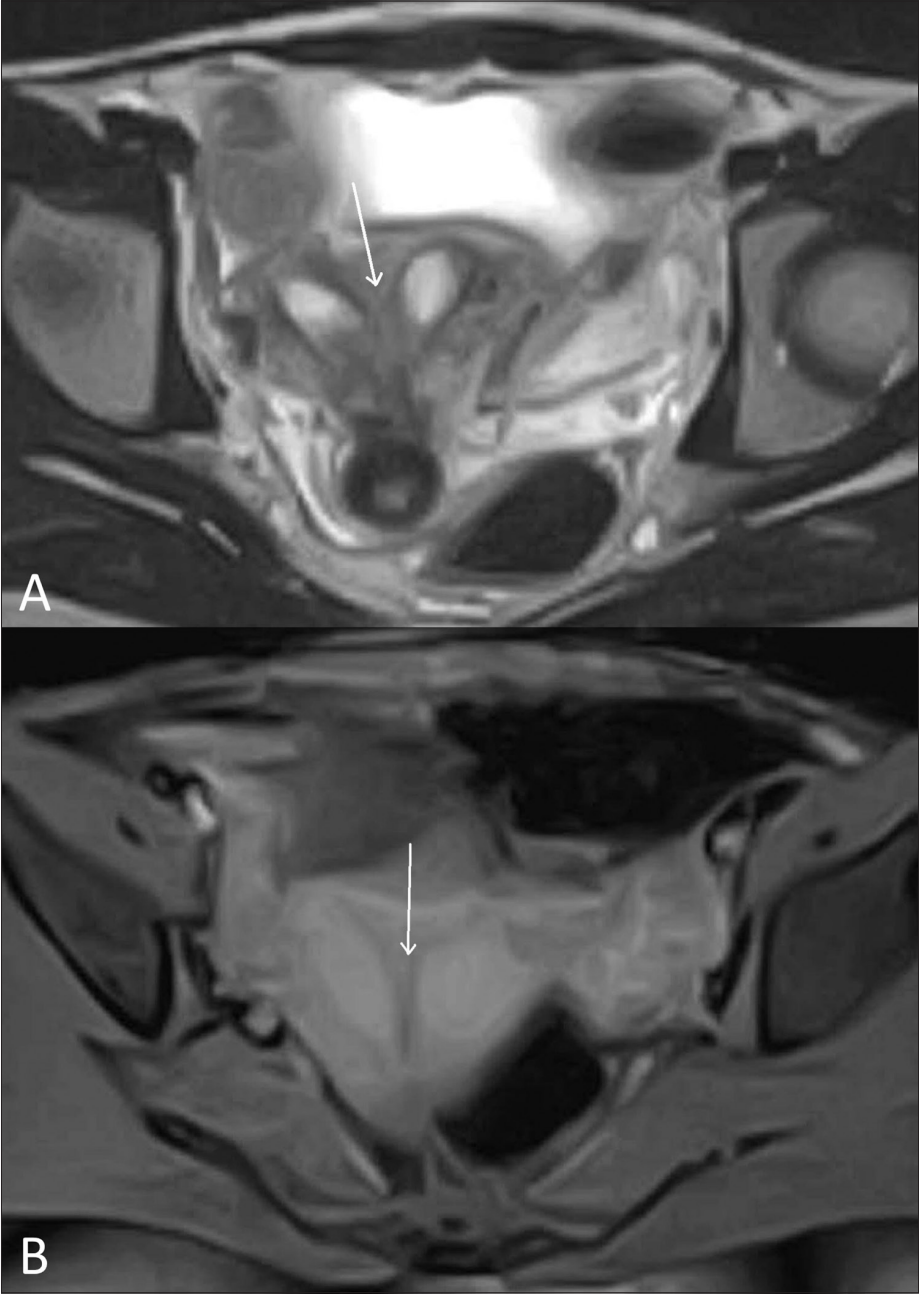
Uterus subseptus. Axial T2W (A) and fat-suppressed T1W (B) MRI images show a uterus subseptus (white arrows show incomplete septum)
